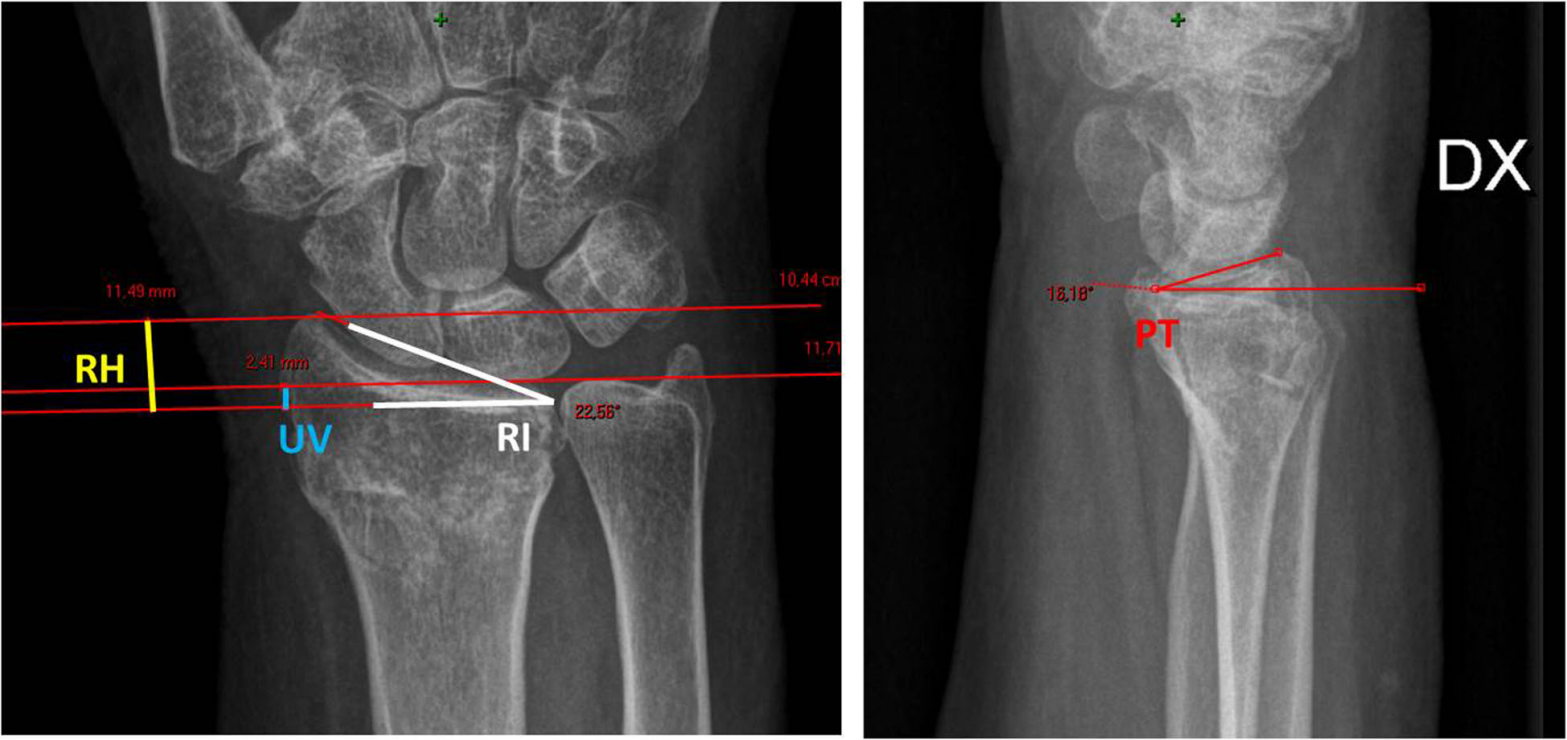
Standard (postero-anterior and lateral view) radiographs showing normal measurements of the distal radius. Palmar tilt (PT) of the radius can be measured by obtaining the angle of intersection between a line drawn tangentially across the most distal points of the radial articular surface and a perpendicular to the midshaft of the radius. Radial inclination (RI) is the angle of the distal radial surface with respect to a line perpendicular to the shaft. Radial height (RH) is the distance between two parallel lines drawn perpendicular to the long axis of the radial shaft, one from the tip of the radial styloid and the other from the ulnar corner of the lunate fossa. Ulnar variance (UV) refers to the relative lengths of the distal articular surfaces of the radius and ulna.
